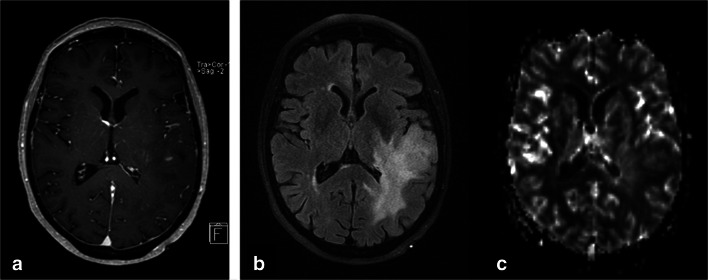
Imaging of a 74-year-old patient with IDH wild-type astrocytomas WHO grade III (FLAIR sequence, a) with only local contrast agent uptake (post-contrast T1-weighted sequence, b), and low tumor perfusion (c, CBV map of DSC-MRI), but tumor progression within 130 days
